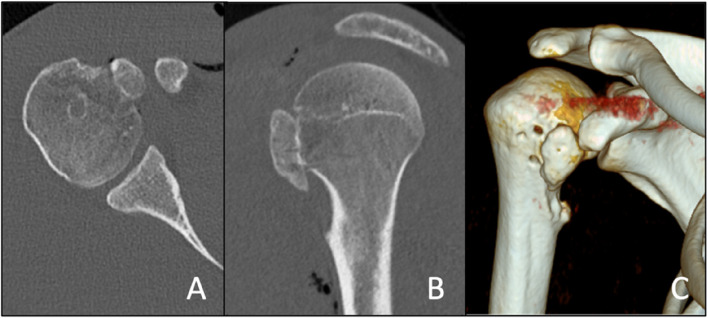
Postoperative axial computed tomography (CT) image in axial (A) and sagittal views (B), and 3D CT image (C)
